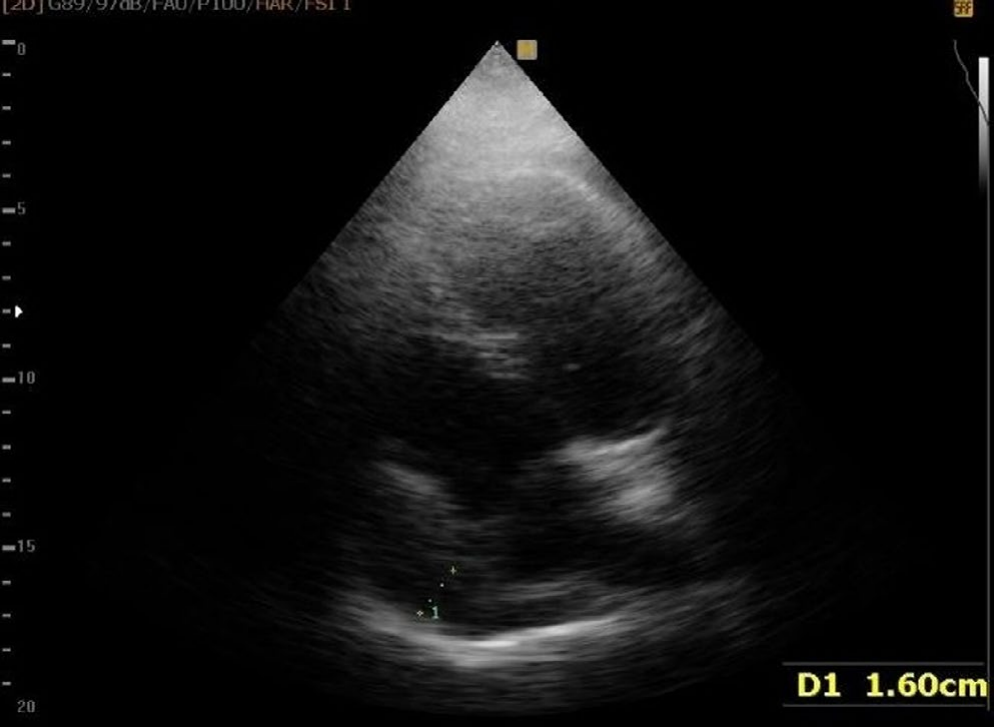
Case 8. Echocardiography shows normal LV size and function, without regional wall motion abnormalities, and a circumferential pericardial effusion without significant respiratory variation on mitral and tricuspid valves
